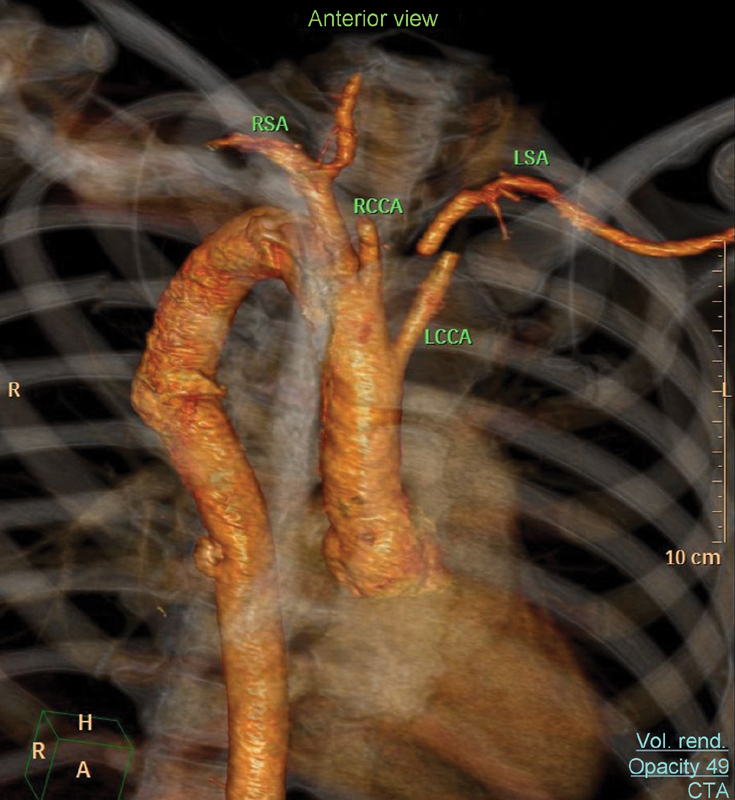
Postoperative computed tomography angiography. Note that the LSA is not connected to the descending aorta and is fulfilled through collaterals. LCCA, left common carotid artery; LSA, left subclavian artery; RCCA, right common carotid artery; RSA, right subclavian artery.
